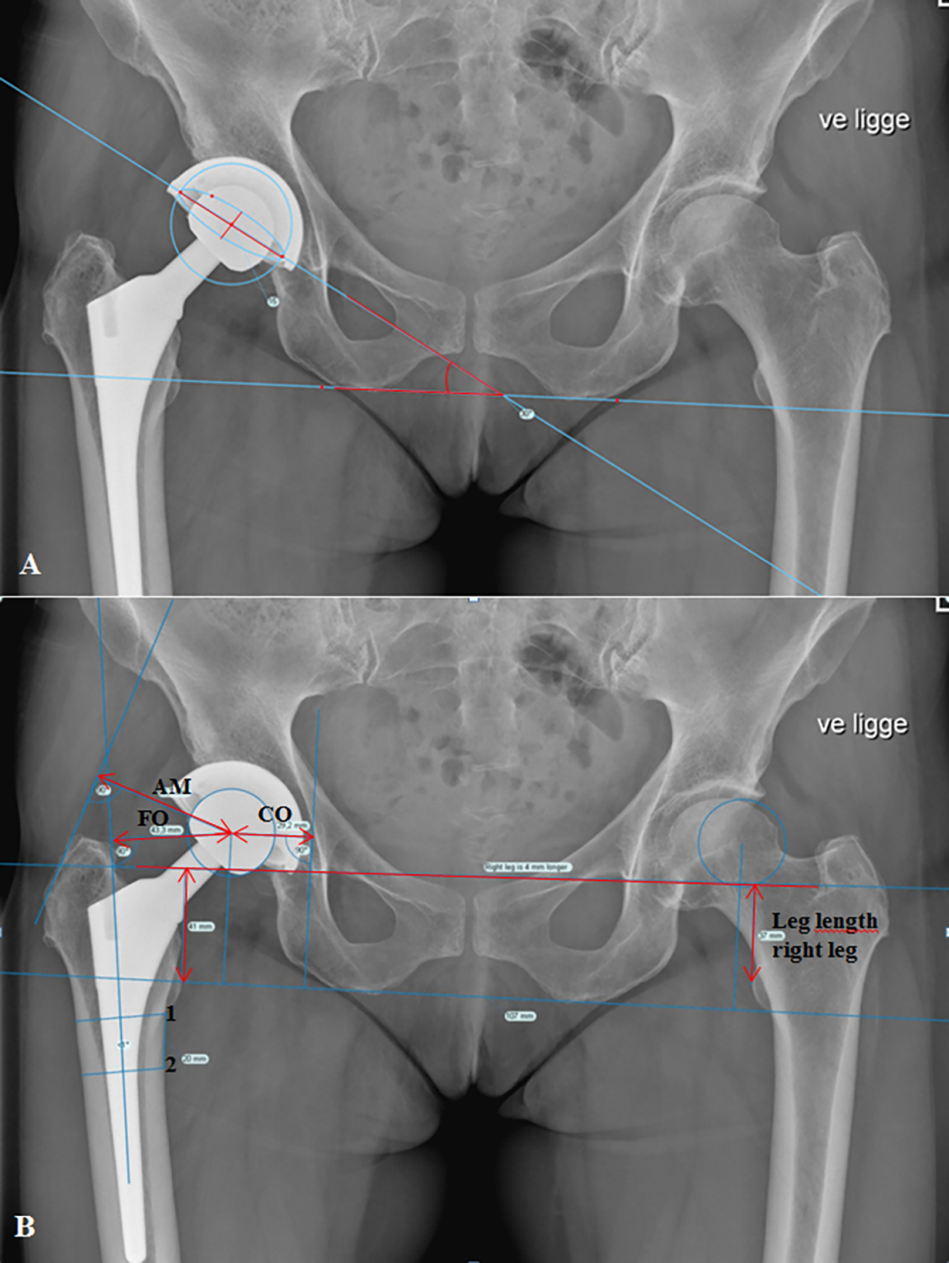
Example of measurements in TraumaCad®. (A) Anteversion and inclination on postoperative anterior-posterior radiographs of the pelvis. (B) Center of rotation (COR) marked on the operated side. The femoral shaft line was drawn from the center of two parallel lines with the shortest length across the femur extracted from two points marked at the distal level of the lesser trochanter and 20 mm distal from the lesser trochanter.
